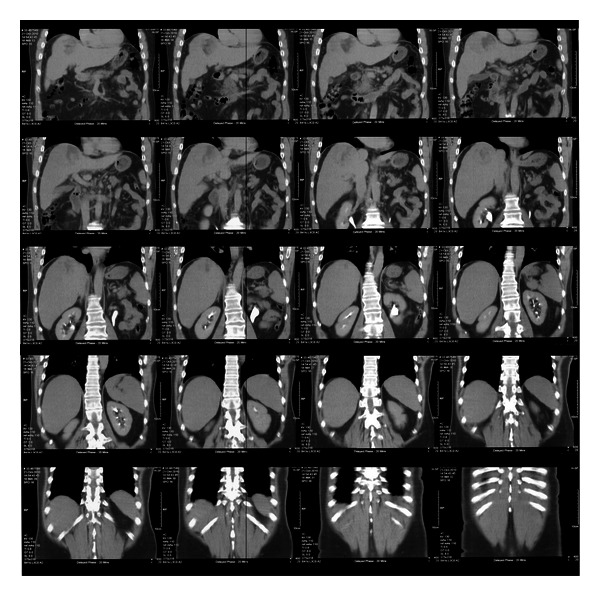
Abdominal CT scan with portovenous contrast showing a lesion in segment 7/8 of the liver.
